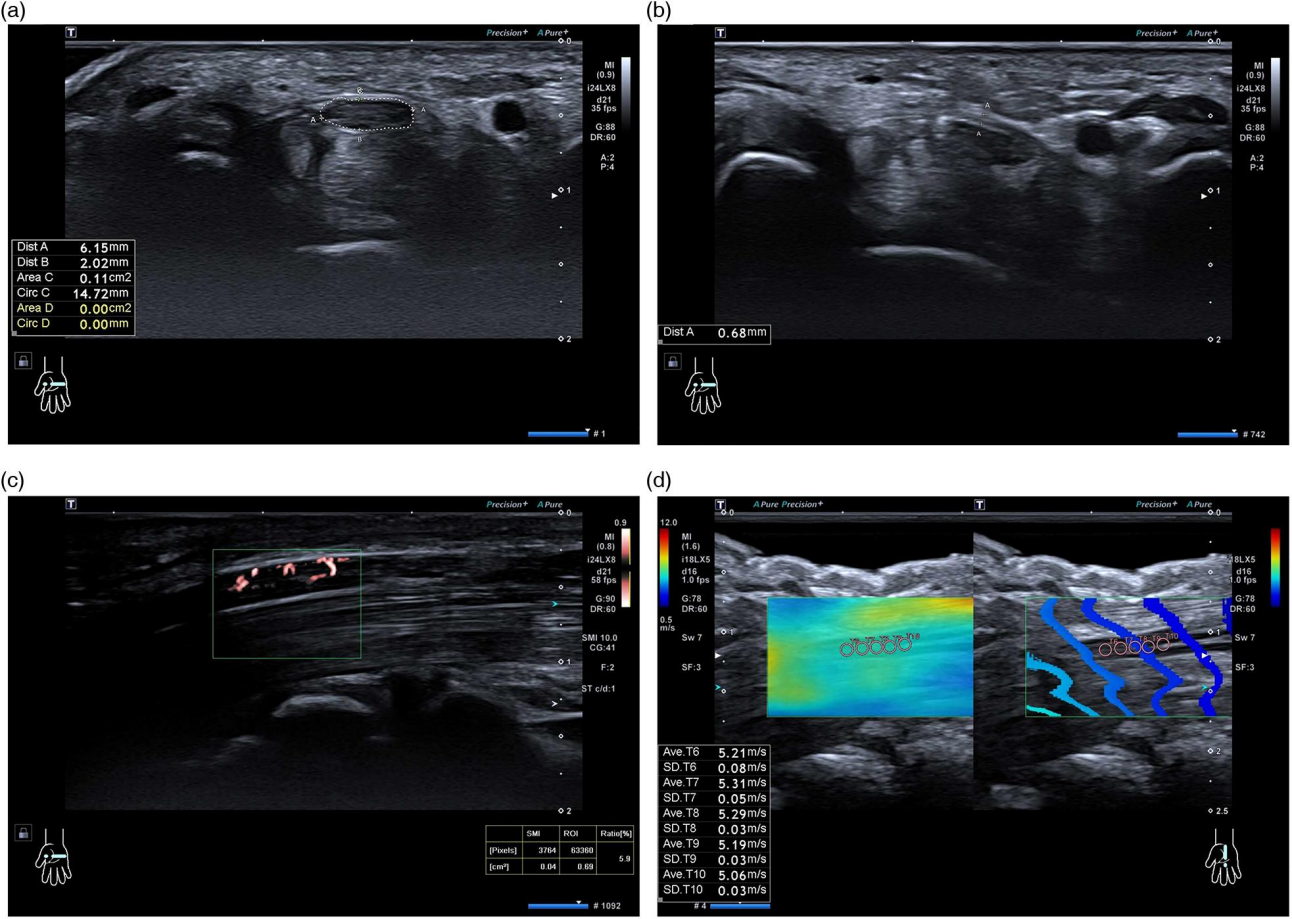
Ultrasound measurement of the median nerve using B-mode, SMI, and SWE techniques. (a) Cross-sectional area (CSA) of the median nerve measured on B-mode ultrasound at the pisiform level. (b) Transverse carpal ligament (TCL) thickness measurement anterior to the median nerve. (c) Superb Microvascular Imaging (SMI) showing intraneural blood flow signals. (d) Shear Wave Elastography (SWE) image displaying a 1-mm region of interest (ROI) within the median nerve.
